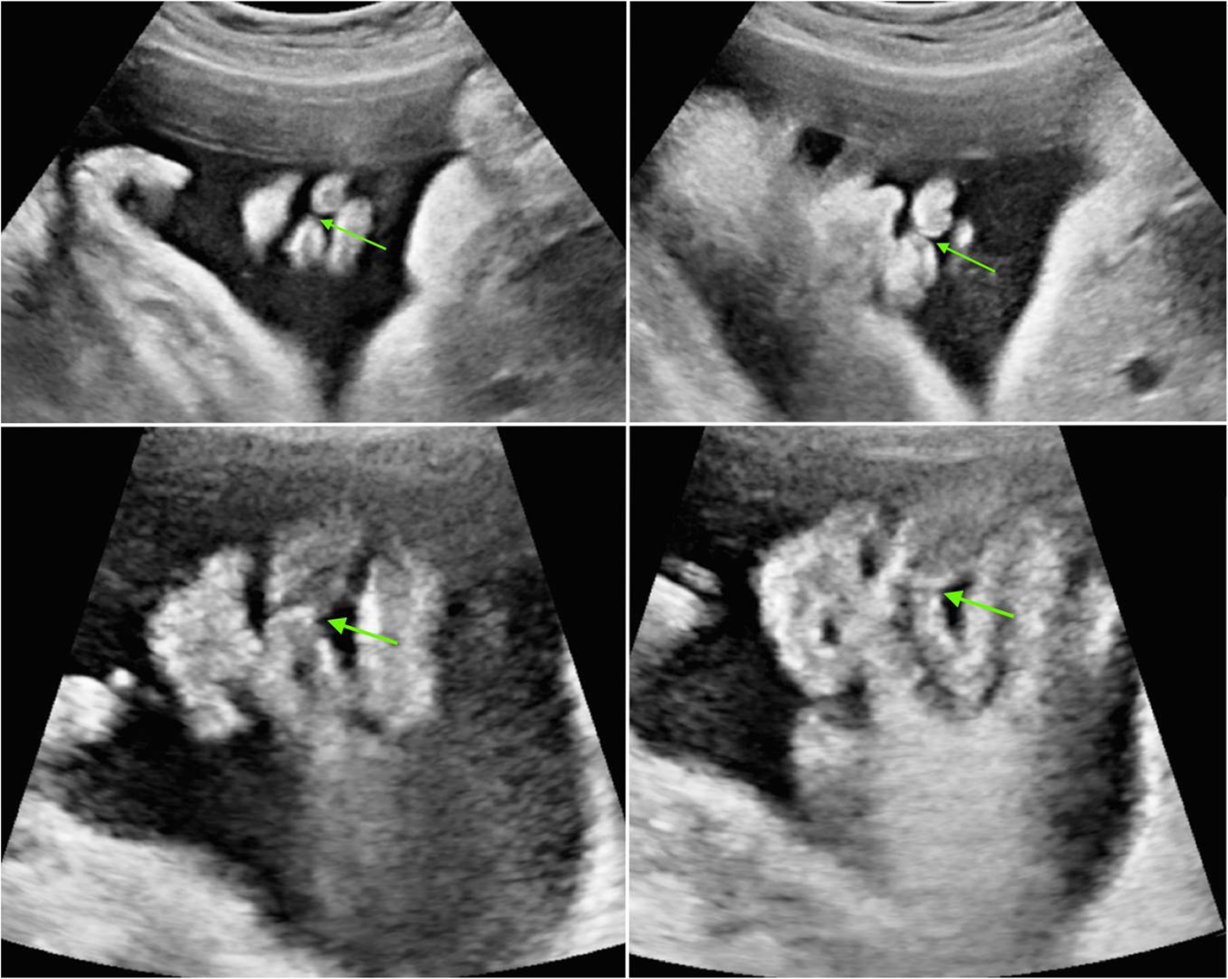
Transabdominal ultrasonography of Case 2, 35 weeks’ gestation. Arrows indicate cleft lip site
